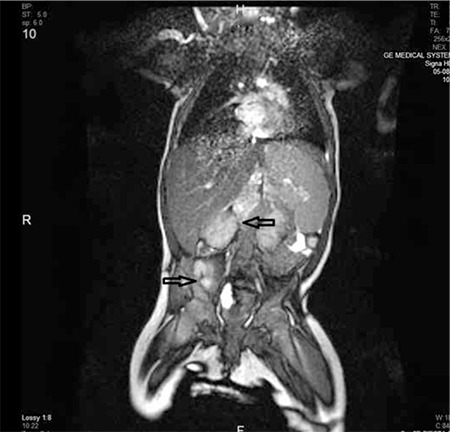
Abdominal magnetic resonance imaging (MRI) with solid mass behind the right psoas major muscle.
